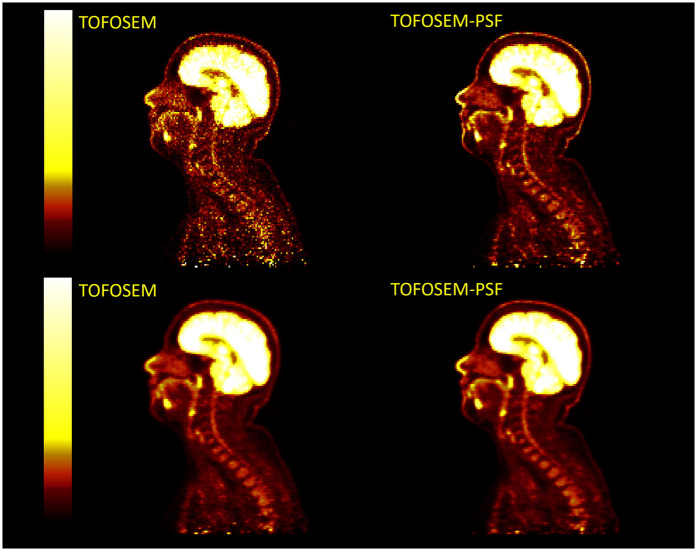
Examples of PET images with post-filtering (5 mm transaxial Gaussian filter and 3 point axial convolution [1 4 1] filter) applied (bottom row) to TOFOSEM reconstructed images with and without PSF modelling, displayed in ${\mathrm{SUV}}_{\mathrm{bw}}$.
